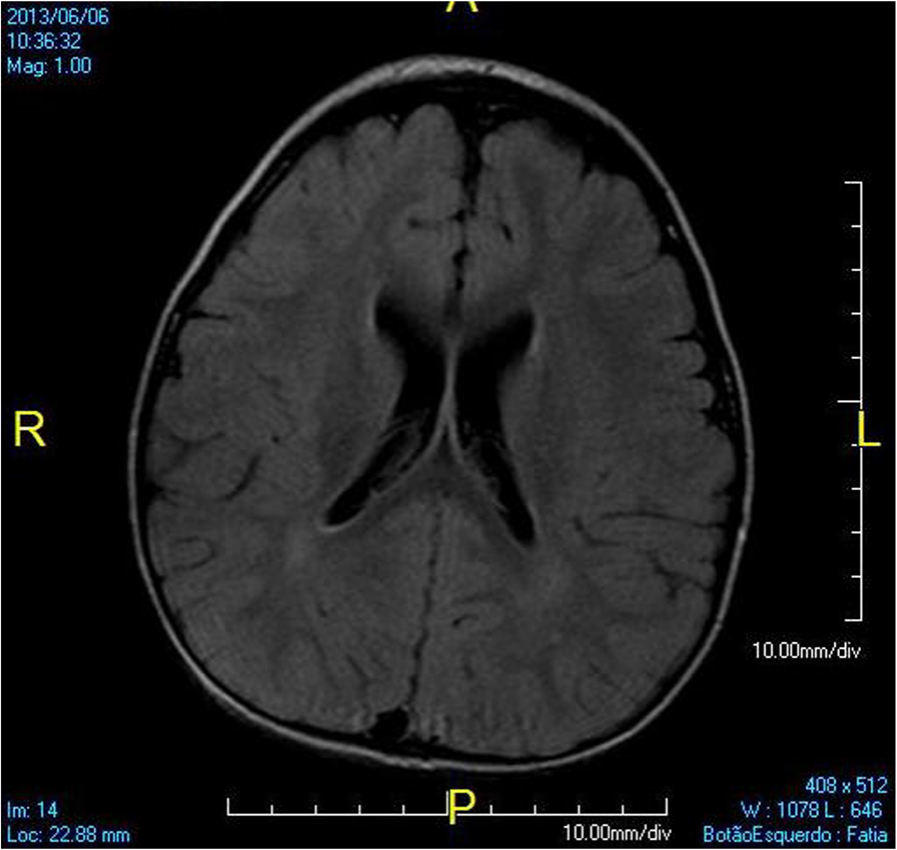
Brain MRI - Increased signal in FLAIR images in the withe matter territories adjacent to the lateral ventricles bodies and subcortical zone
